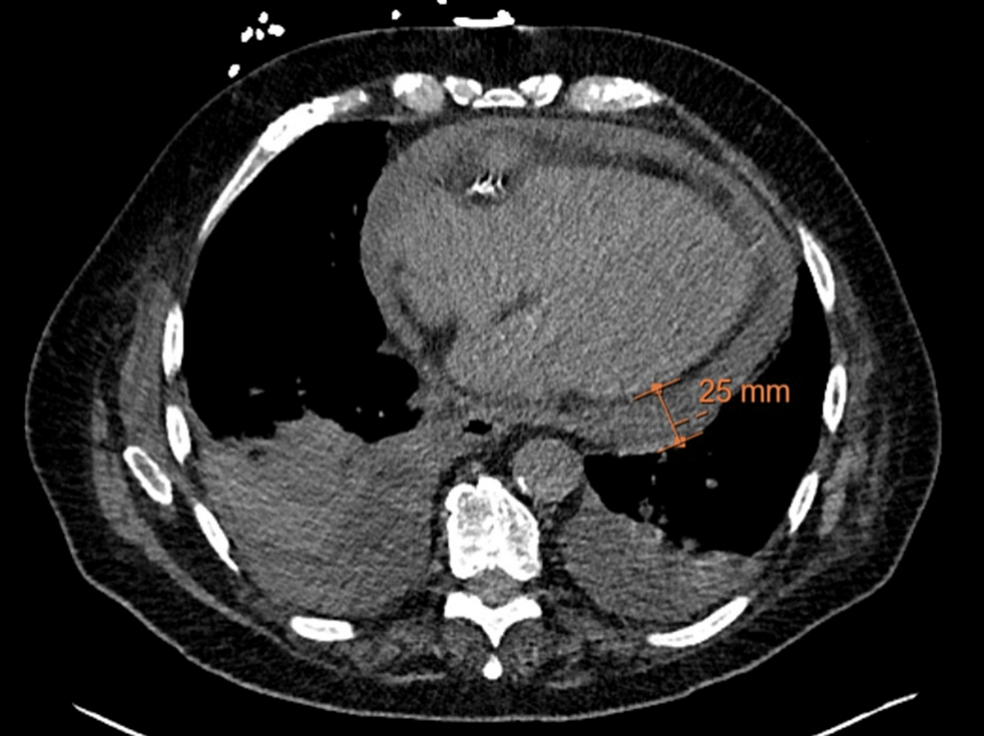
CT chest axial view demonstrating a moderate to large pericardial effusion and bilateral pleural effusions
